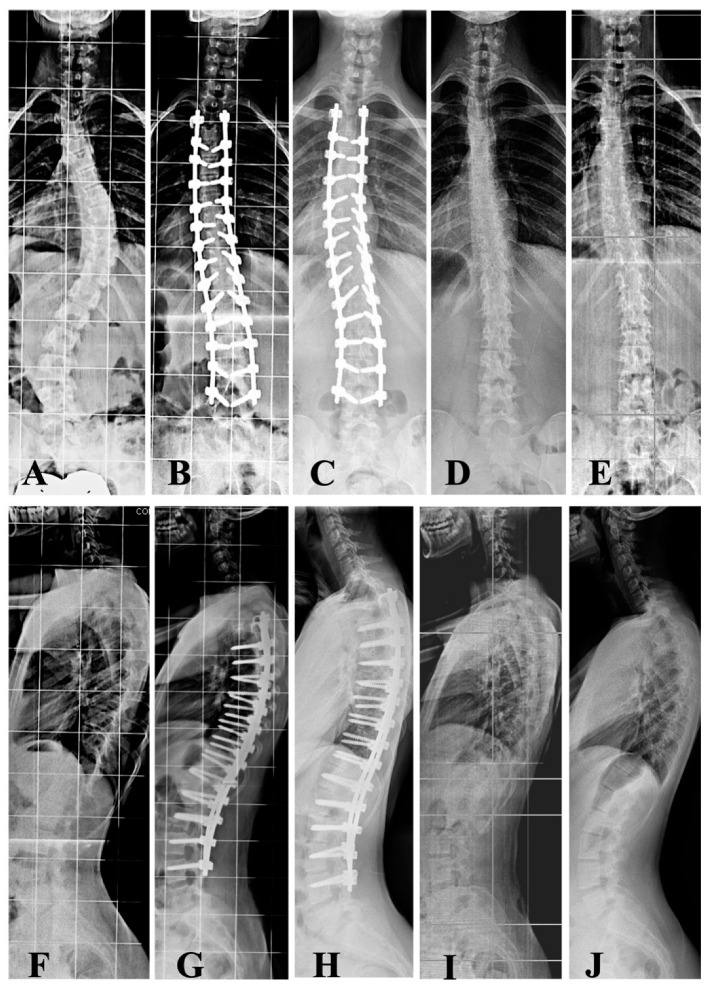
A 13-year-and-2-month-old girl with Lenke type 1BN AIS (50°/36°) with Risser 1 (A) and thoracic hypokyphosis of 13° (F), was surgically treated by a posterior approach involving T3-L4 instrumentation without fusion with slight hypercorrection of both curves (B). Three years and two months after the initial surgical intervention and just before the removal of instrumentation, the main and compensatory curves remained unchanged towards the opposite side of the initial curves (C). The radiograph after removal of the vertebral implants shows minimal residual scoliosis towards the opposite side of the curves that existed before surgical treatment (D). These minimal scoliotic curves remained unchanged on the last radiograph, taken two years after the removal of the instrumentation (E). Concerning the sagittal plane, the postoperative radiograph after surgical correction demonstrated an increase in previous hypokyphosis (G), which remained unchanged in the radiograph prior to removal of the instrumentation (H), after its removal (I), and in the last X-ray check-up two years after instrument removal (J).
